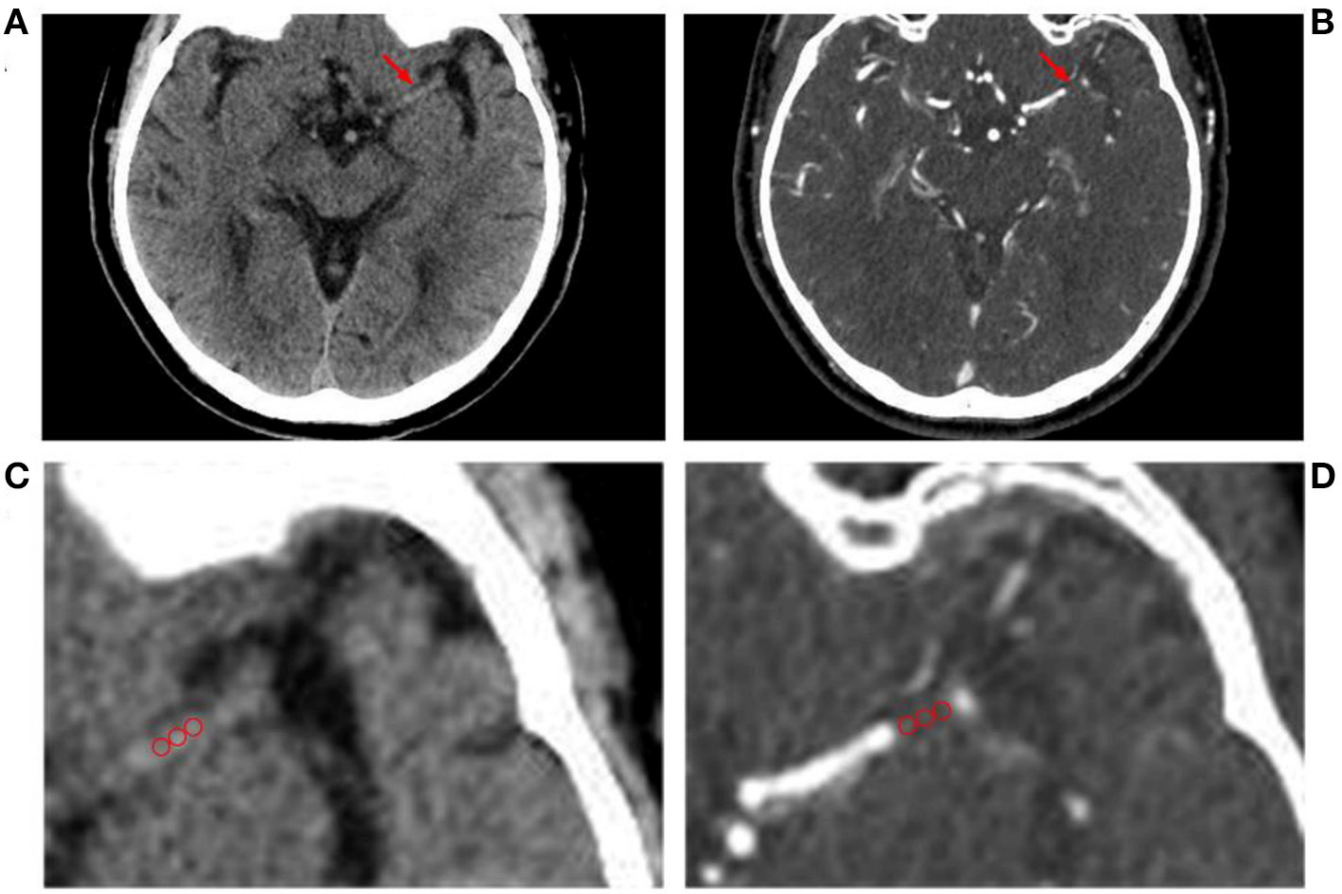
Measurement of thrombus permeability on non-contrast CT (nCT, A&C) and CT angiography (CTA, B&D). Three regions of interest (red circles) are placed in the proximal, middle, and distal parts of the thrombus (red arrow) to calculate the mean Hounsfield unit (HU) density value of the occlusion site. (C,D) Are partial magnifications of (A,B).
